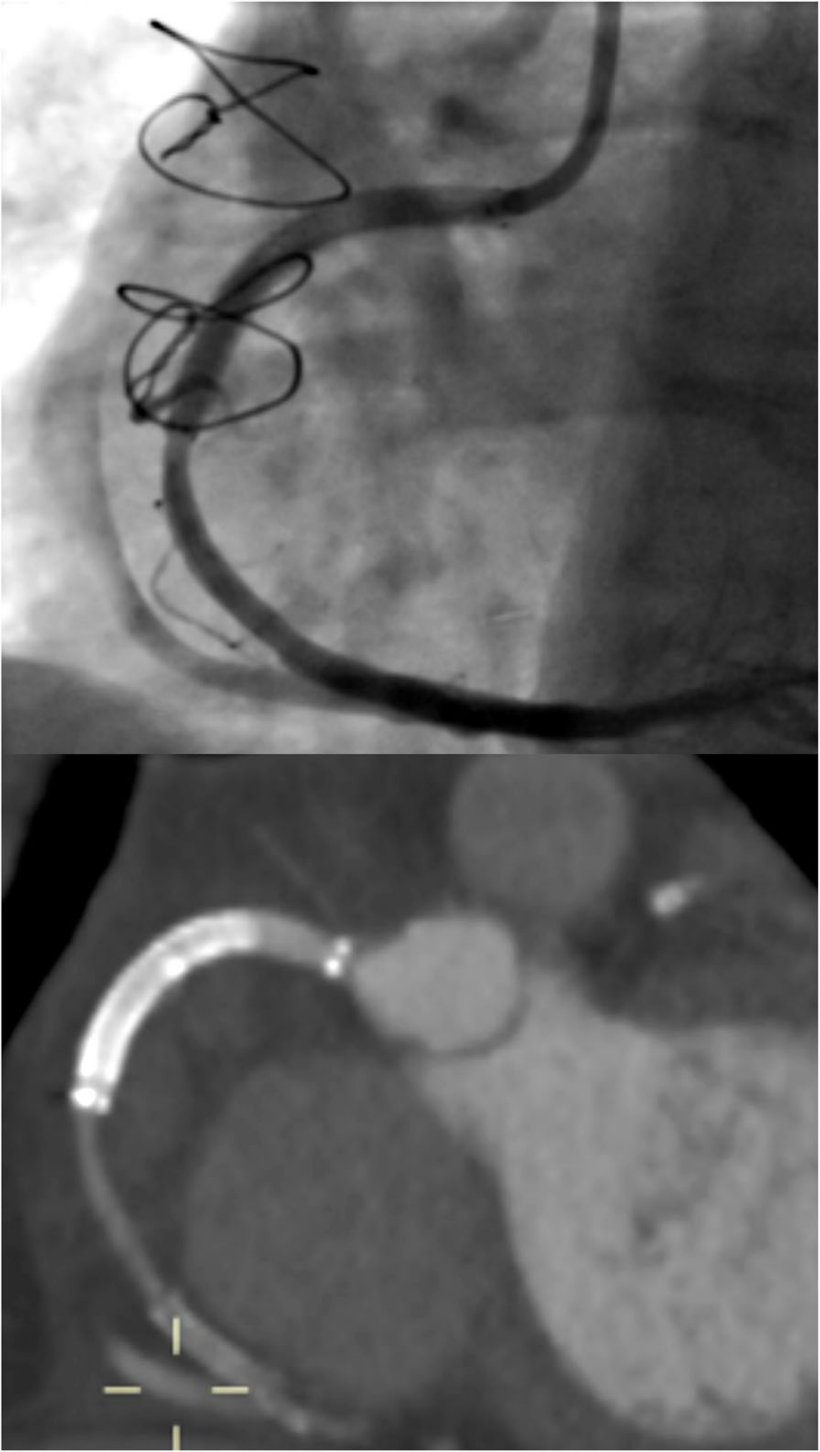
AP projection and CT coronary angiogram showing the Viabahn stent graft in situ with distal patency (reflux of contrast seen into the right SVG)
